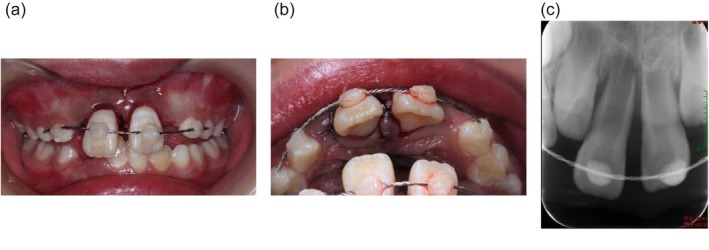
Post‐surgical intraoral and radiograph views of resin splinting. (a) Labial view showed resin splint; (b) occlusal view showed resin splint treatment with resin splint; (c) radiograph confirming resin splint placement.
